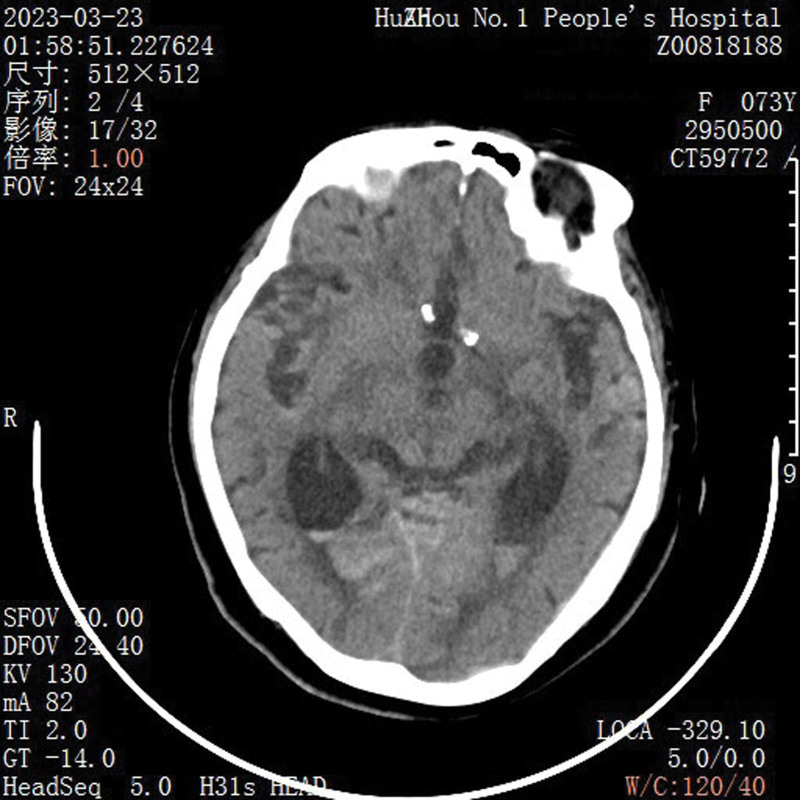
CT taken after the patient’s pupil changed, no evidence of hemorrhage or cerebral herniation. CT = computed tomography.
